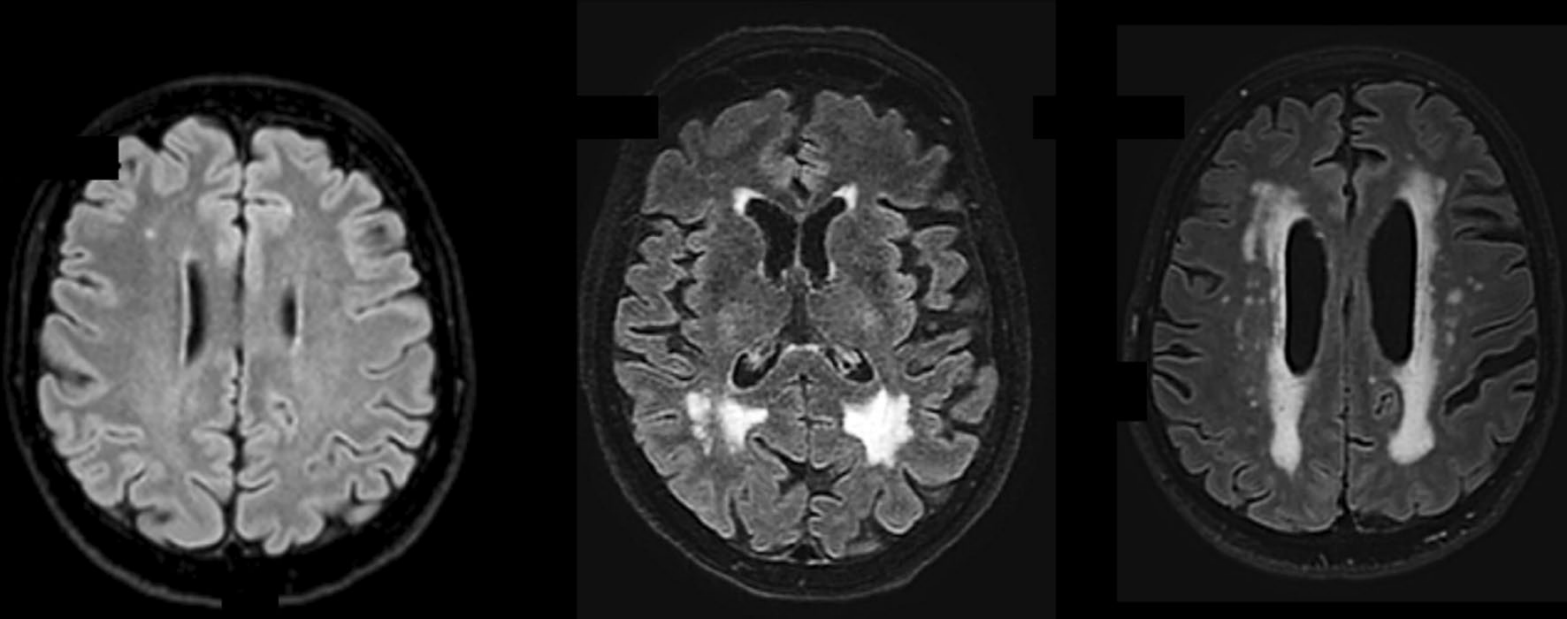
Axial FLAIR images. On the left, one punctate lesion on the right frontal white matter (Fazekas 1); in the middle, confluent foci at retrotrigonal white matter; on the right, diffuse and confluent subcortical, peri and paraventricular white matter lesions
